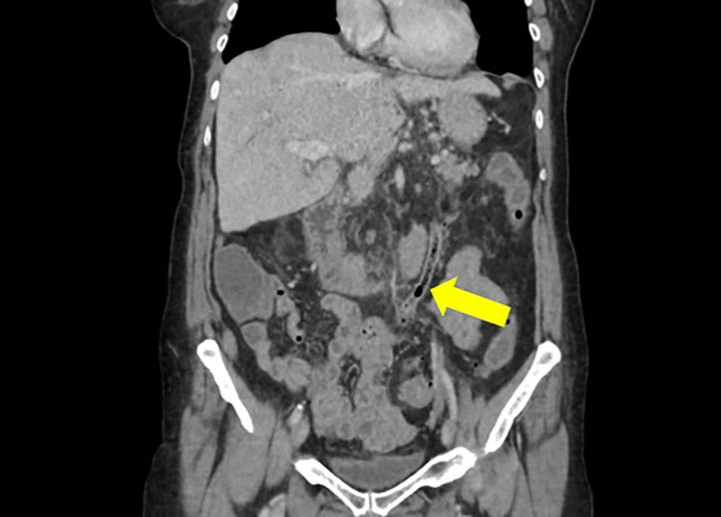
Coronal section of non-contrast CT scan demonstrating the colovenous fistula between the inferior mesenteric vein and sigmoid colon (yellow arrow); air is seen tracking within the lumen of the inferior mesentericvein.
